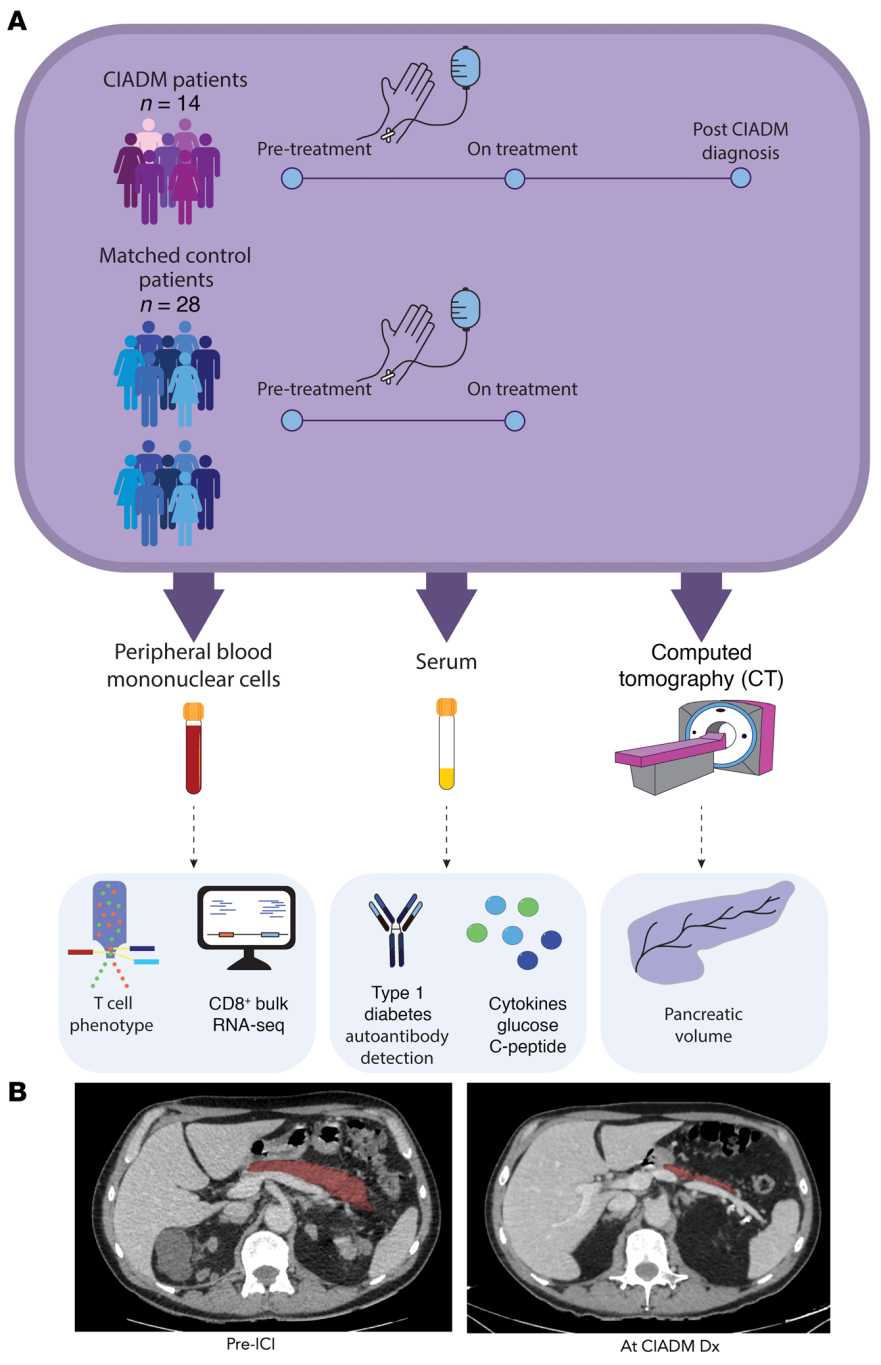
(A) Summary of methodology. (B) Representative CT scans of a patient with CIADM prior to ICI therapy and at the time of CIADM diagnosis (red indicates the pancreatic area).
